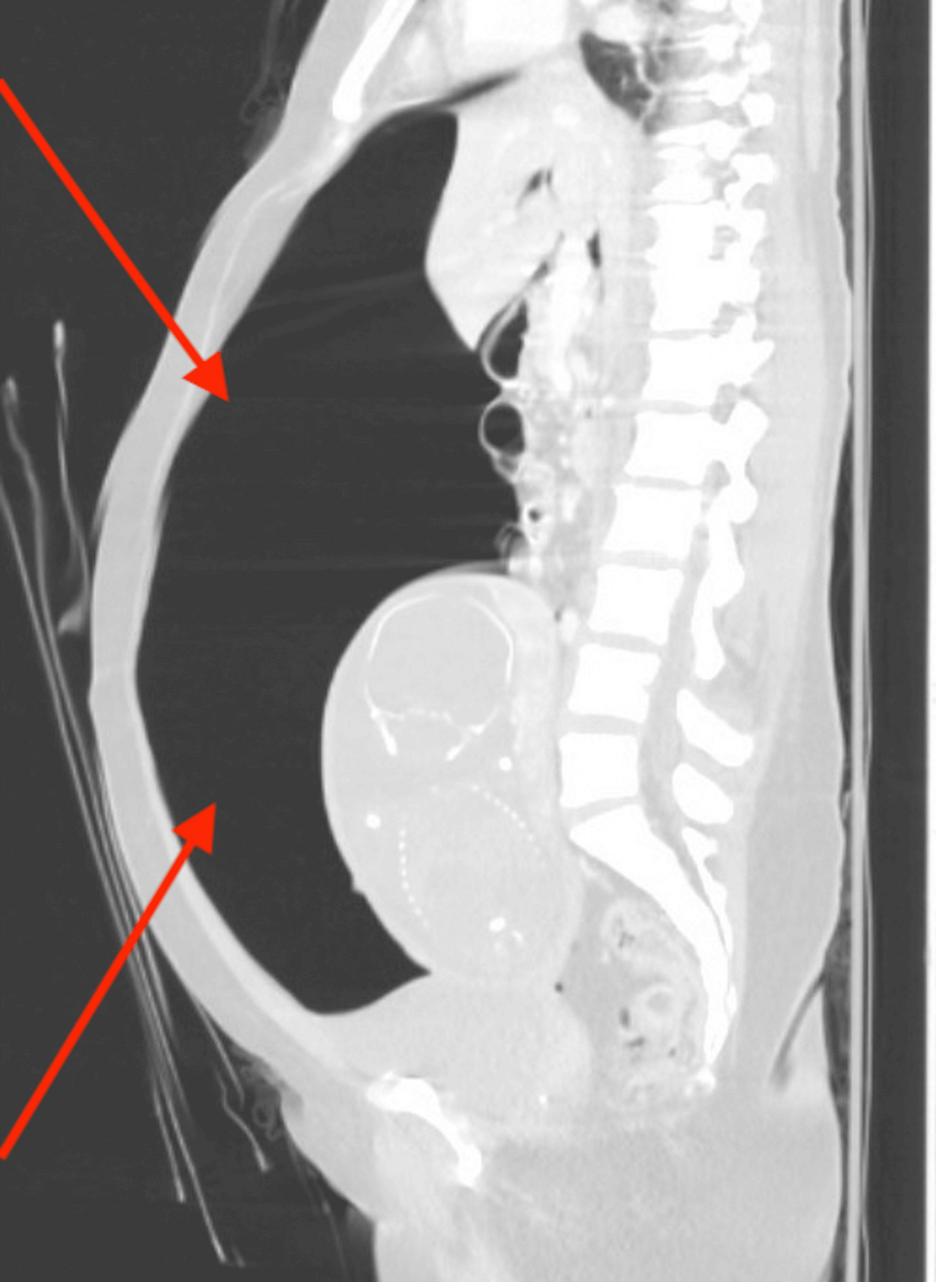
Sagittal View CT scan With Intravenous Contrast of Patient Prior to Laparotomy (Lung window to Further Highlight Significant Free Air in the Abdominal Cavity, Indicated by Two Arrows)
